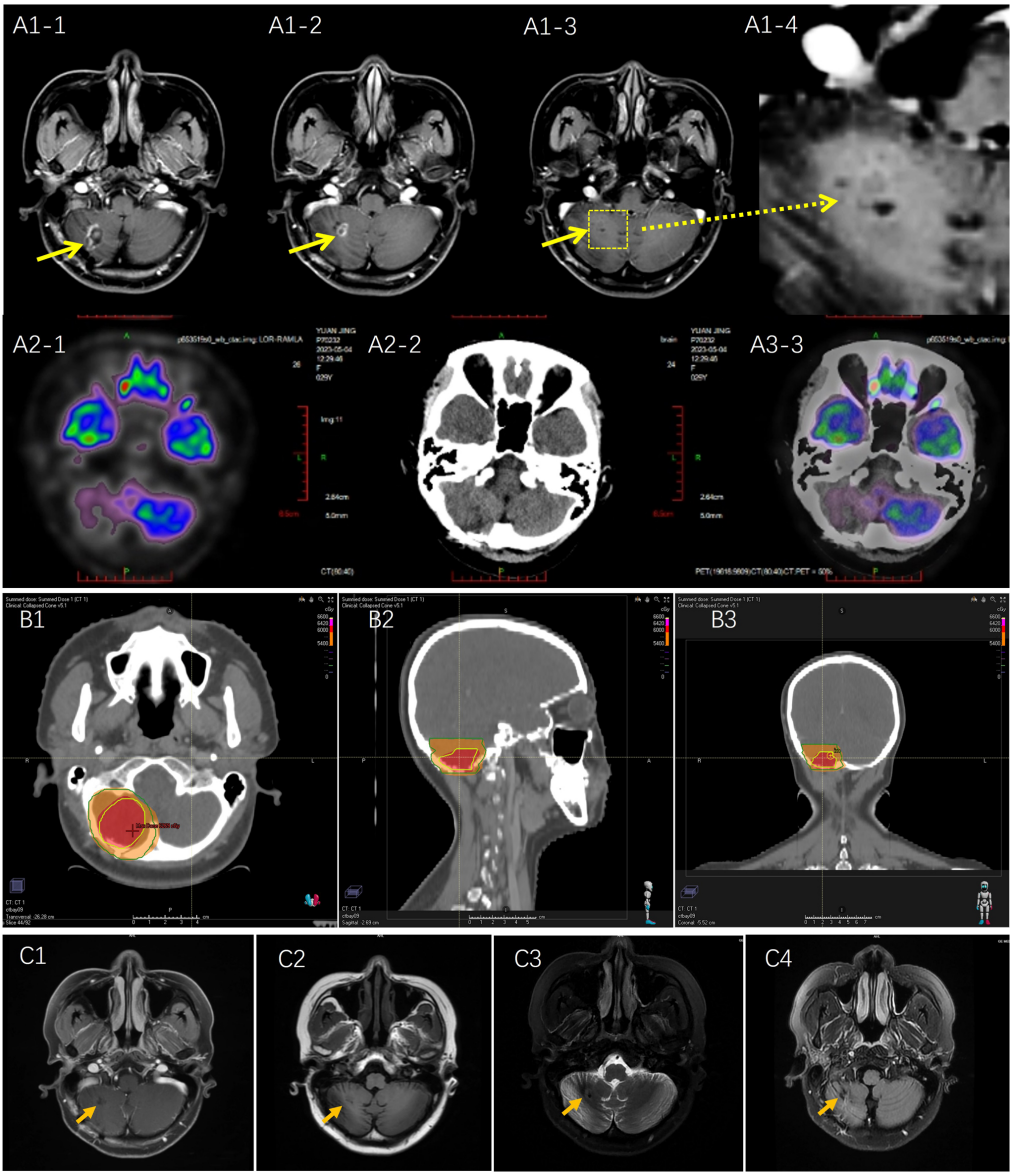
(A) Postoperative Magnetic resonance imaging (MRI) and Positron Emission Tomography (PET) scan on April 2023: (A1) MRI scan: long T1 and T2signal shadows were observed with annular enhancement; (A2) PET scan no metastasis and recurrent tumor were detected. (B) Radiotherapy planning: According to RTOG radiotherapy standard, tumor bed as the gross target volume (GTV) was delineated based on MRI T1 enhanced image and T2 Flair image, including postoperative area and edema area, and expanding the GTV range by 2.0 cm became clinical target volume (CTV). Red area: GTV was at a dose of 60 Gy; Orange area: CTV area was at a dose of 54 Gy. (C) Brain MRI scan was performed on October 11, 2023.No new recurrent lesion was detected. (C1) T1 enhancement; (C2) T1 FLAIR; (C3) T2 propeller; (C4) T2 FLAIR.
